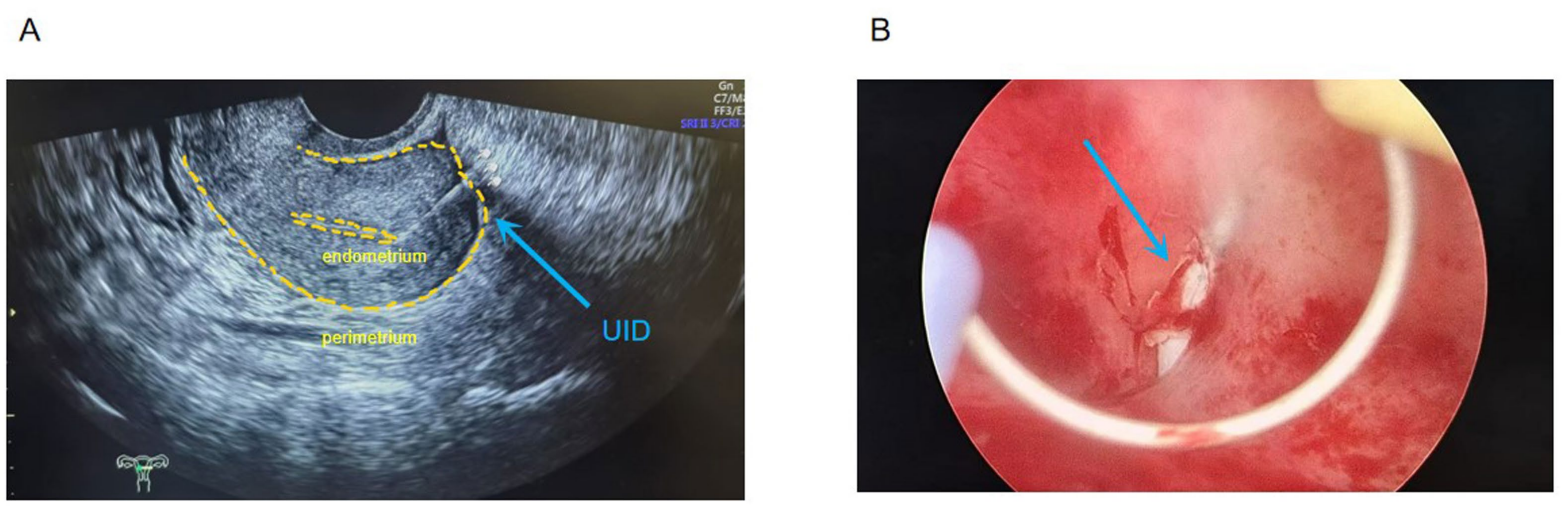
(A) Preoperative transvaginal ultrasound showing IUD echogenicity with partial protrusion toward the serosal surface (arrow). (B) Hysteroscopic view showing the IUD embedded within the myometrium near the left uterine horn (arrow).
